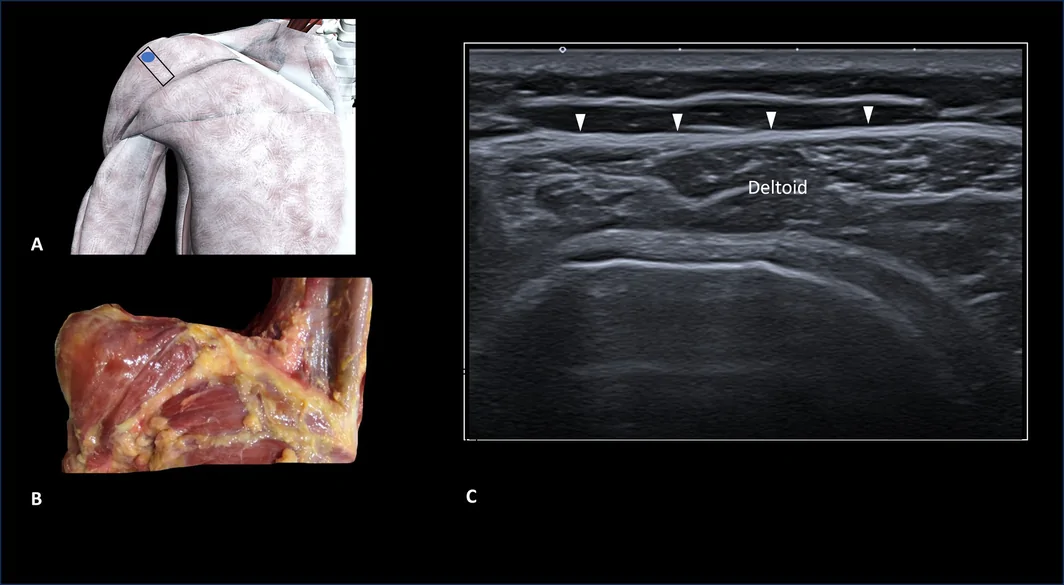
Deltoid fascia. A, Probe positioning. B, Anatomical dissection showing the deltoid fascia as a dense fibrous layer enveloping the deltoid muscle, continuous with the clavipectoral fascia proximally and the brachial fascia distally. C, Ultrasound image (*transverse view*) displaying the deltoid fascia as a hyperechoic linear structure superficial to the deltoid muscle, with well‐defined fascial‐muscular interface. Arrowheads: deltoid fascia.
